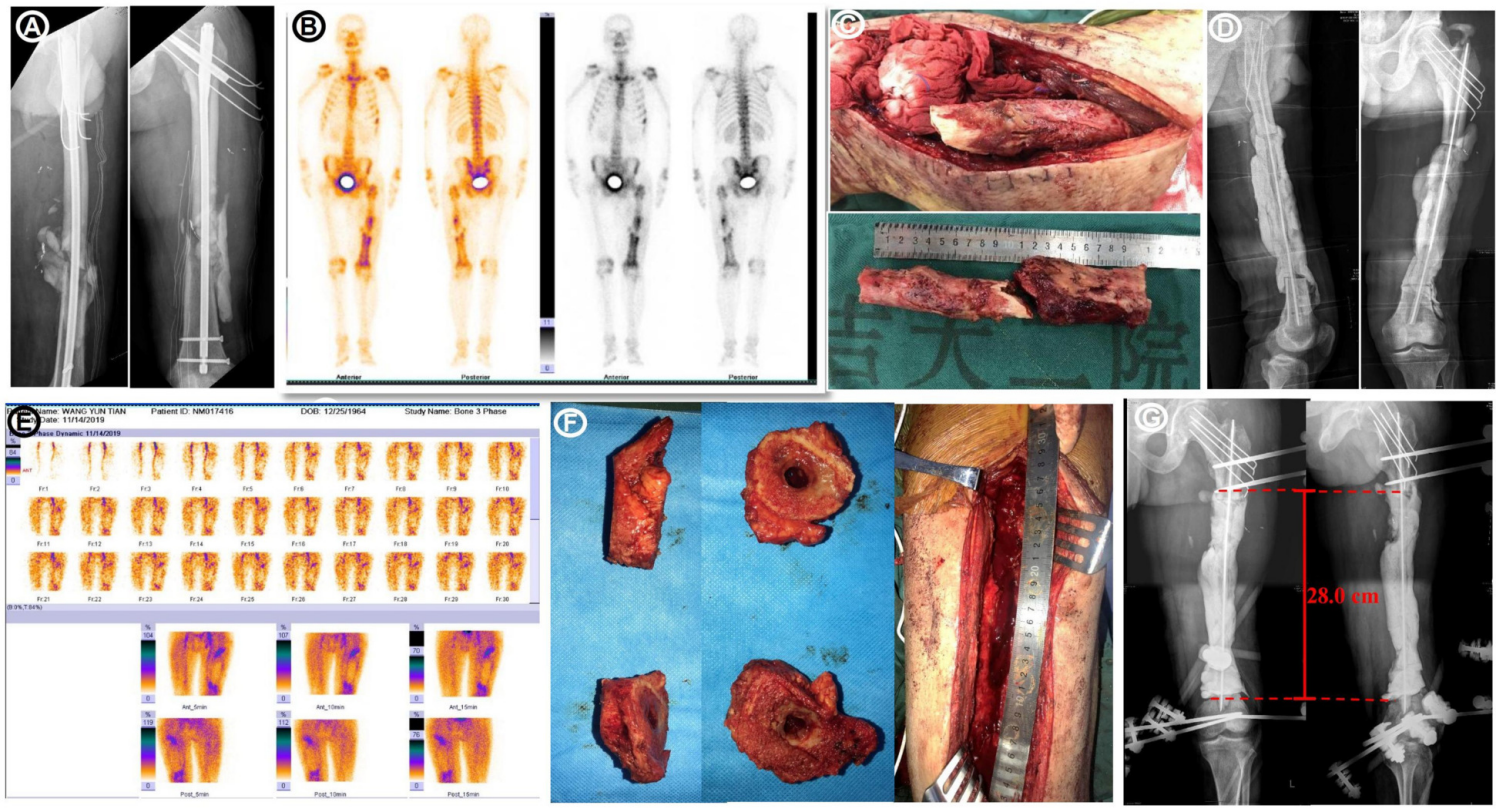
Images of the first and second surgeries. (A) Radiographs obtained before the first debridement. (B) SPECT/CT results showed positive blood flow, pooling, and delayed phases. (C) A large infection at the fracture end was observed during the second debridement and surgical resection of 20 cm of the infected bone. (D) Post-resection and antibiotic-loaded PMMA rod imaging. (E) Before the third debridement, SPECT/CT results indicated positive blood flow, pool, and delay phases. (F) Surgical resection of 8 cm of the infected bone. (G) Radiographs obtained after second debridement.
